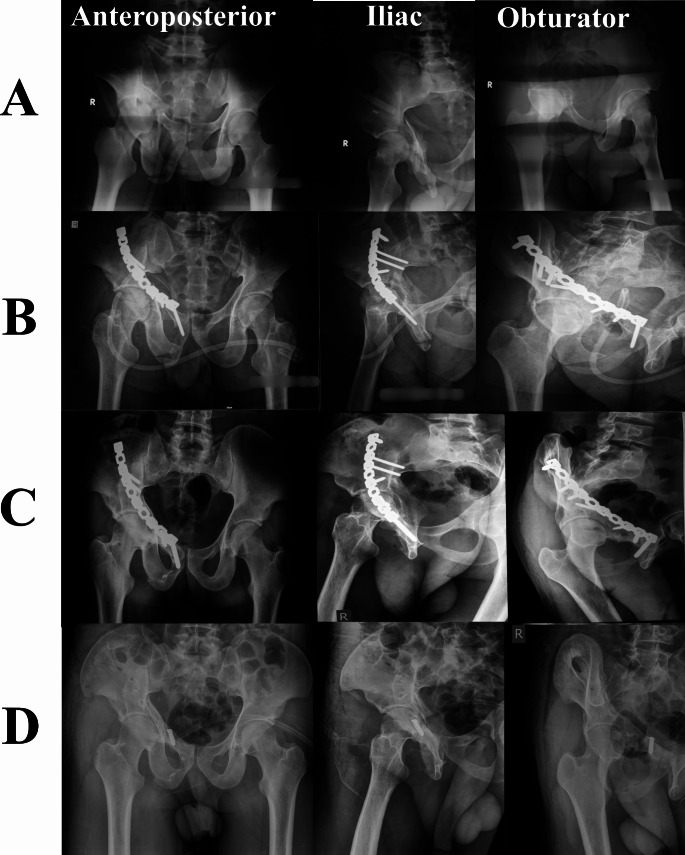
23-year-old male patient who had a T-type fracture treated by ORIF, hardware was performed through a modified Stoppa approach and a lateral window after 30 months of the index surgery. A, plain radiographs of the index injury. B, follow up after ORIF. C, pre-hardware removal. D, after hardware removal. (Patient No. 3 in Supplementary File 2)
